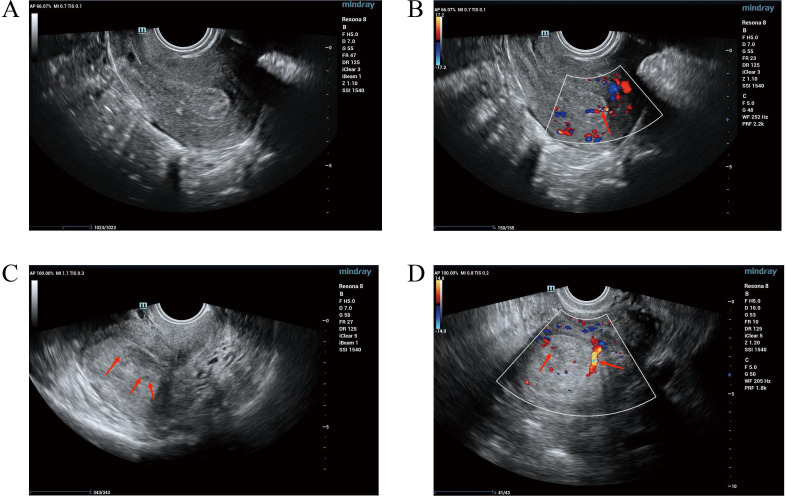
Representative ultrasound images of endometrial lesions. (A) Ultrasound image of a patient with endometrial thickening in the control group, showing a clear boundary between the endometrium and myometrium; (B) Corresponding color Doppler image of the same patient, demonstrating a strip-like blood vessel with Adler blood flow grade I; (C) Ultrasound image of a patient with endometrial carcinoma, showing an indistinct boundary between the endometrium and myometrium of the posterior uterine wall; (D) Corresponding color Doppler image of the same endometrial carcinoma patient, showing disordered and abundant blood flow with Adler blood flow grade II.
